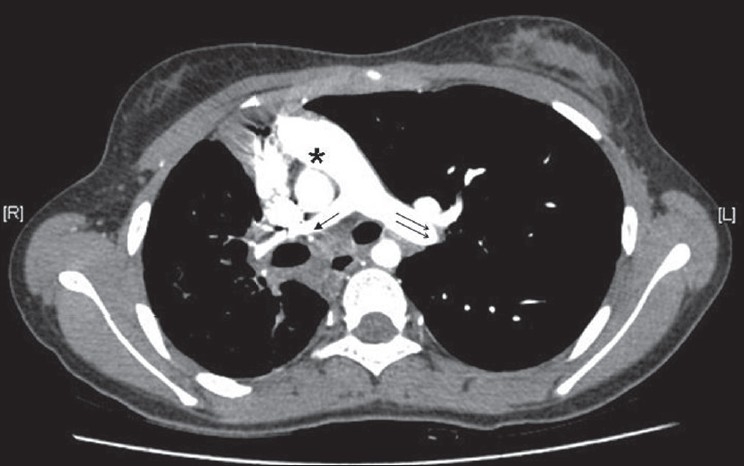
CT thorax showing dilated main pulmonary (asterisk) with normal looking left pulmonary artery (double arrow), and hypoplastic right pulmonary artery (single arrow)
